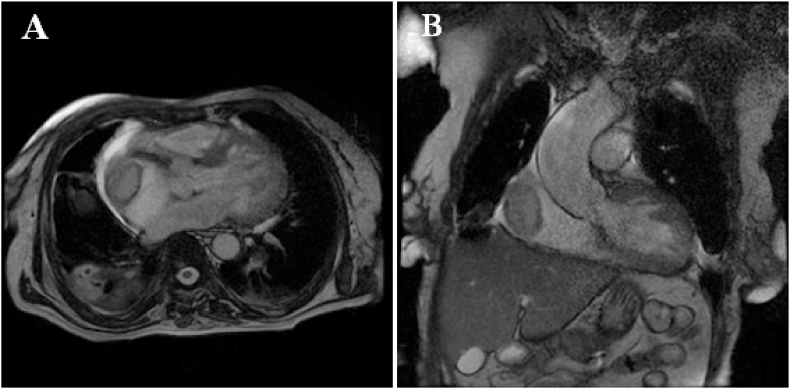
Cardiac magnetic resonance imaging showing a huge thrombus in the right atrium.
